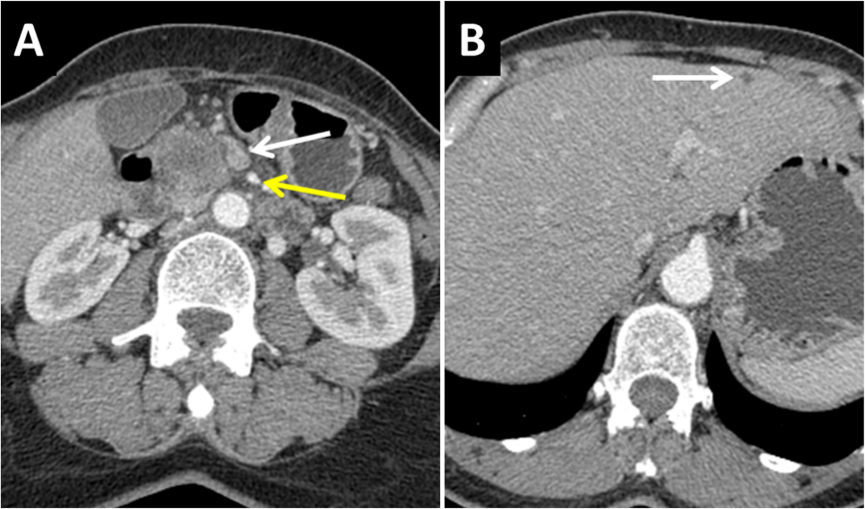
Computed tomography (CT) scan of patient number 3 before initial palliative bypass procedure showing a tumor of the head of the pancreas (T) with lack of invasion of superior mesenteric vein (SMV) (white arrow) and superior mesenteric artery (SMA) (yellow arrow) (A) but unrecognized subcapsular liver metastases (white arrow) (B).
